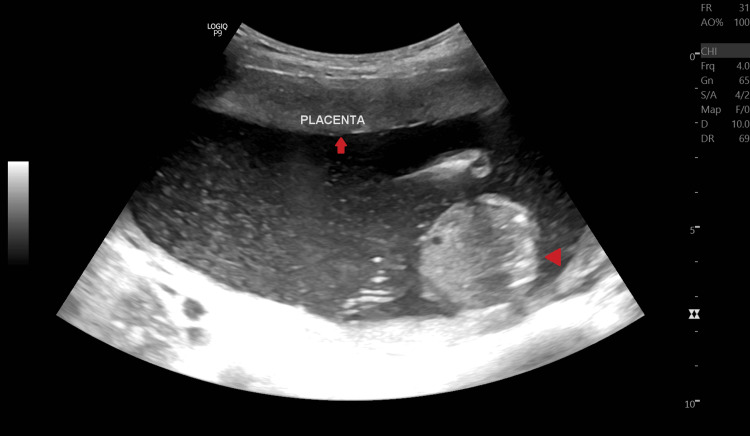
B-mode ultrasonography image showing the position of the placenta on the anterior part of the uterus. The solid arrow (⬆) shows the placenta on the anterior uterine wall, and the arrowhead(◀) shows the fetal abdomen in the axial view.
